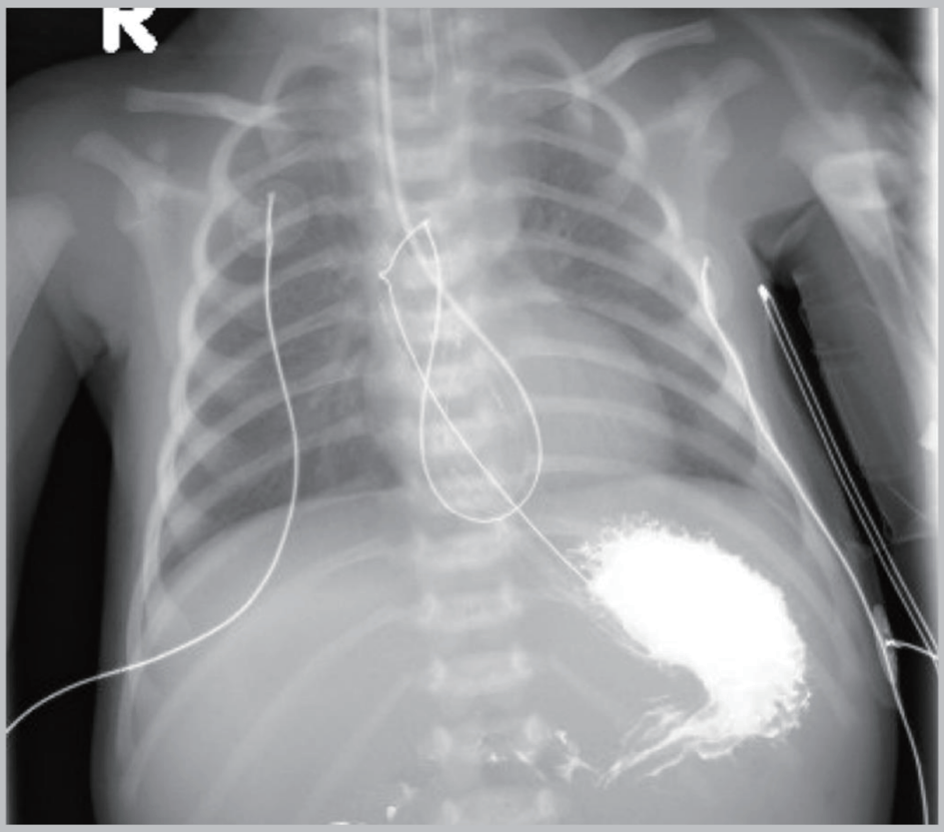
Initial intraoperative chest radiograph revealing looping of the nasogastric (NG) tube within the esophagus and its tip in the fundus of the stomach.
